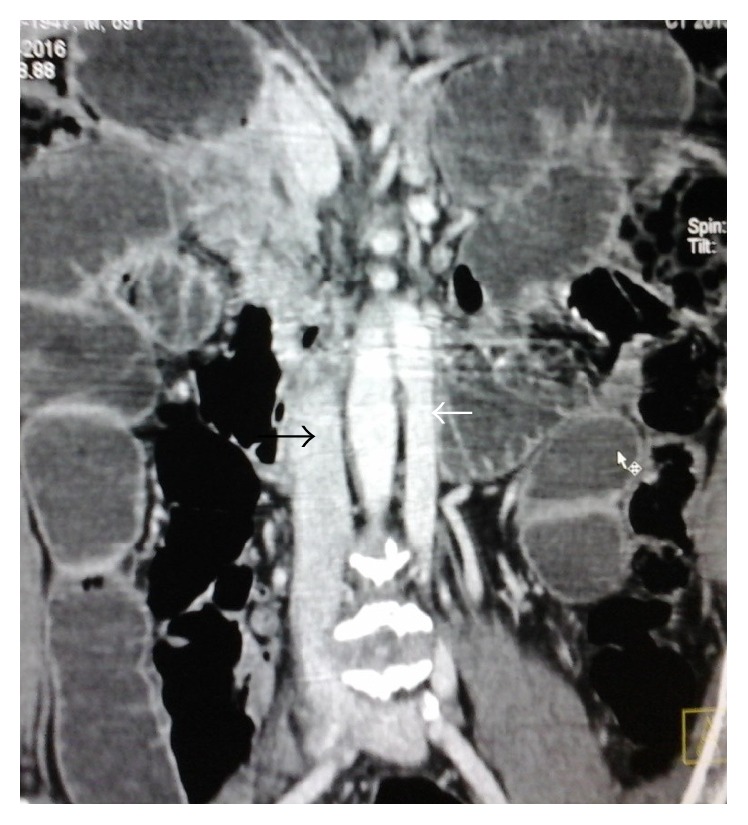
Coronal CT image showing double IVC with small left IVC (white arrow) and large caliber right IVC (black arrow).
